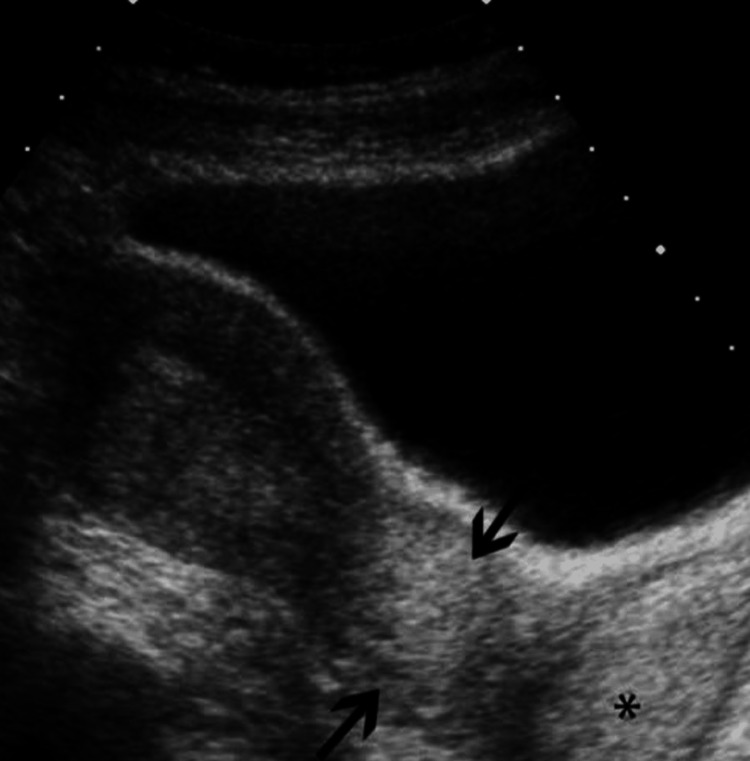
Ultrasonography showing bulky uterus with thickened lower uterine segment and cervix The arrows show a thickened lower segment of the uterus, and the asterisk shows a thickened cervix.
